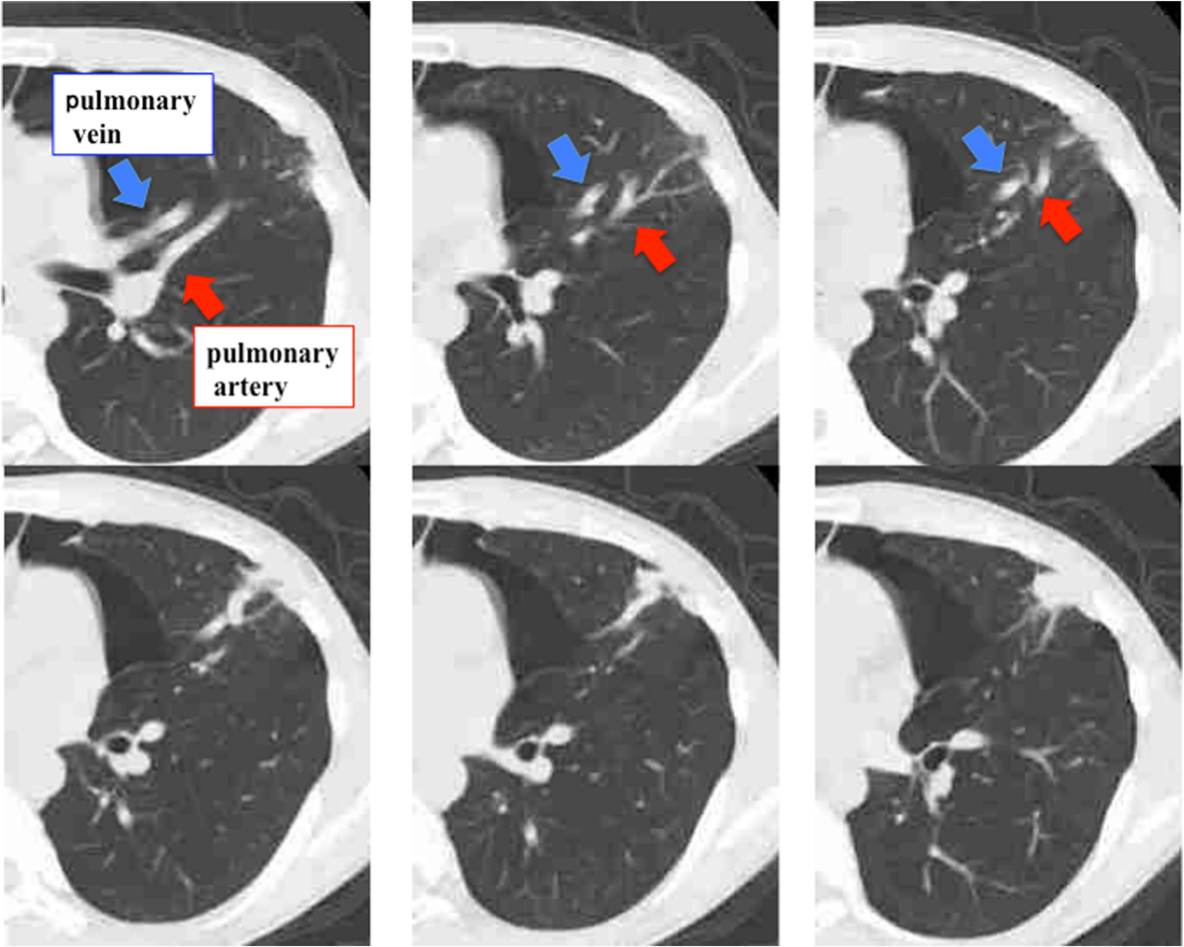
Preoperative non-enhanced chest CT scan findings. The abnormally dilated pulmonary artery and pulmonary vein in the lingular segment run towards the chest wall scar remaining at the site of surgical port placement during the previous operation
